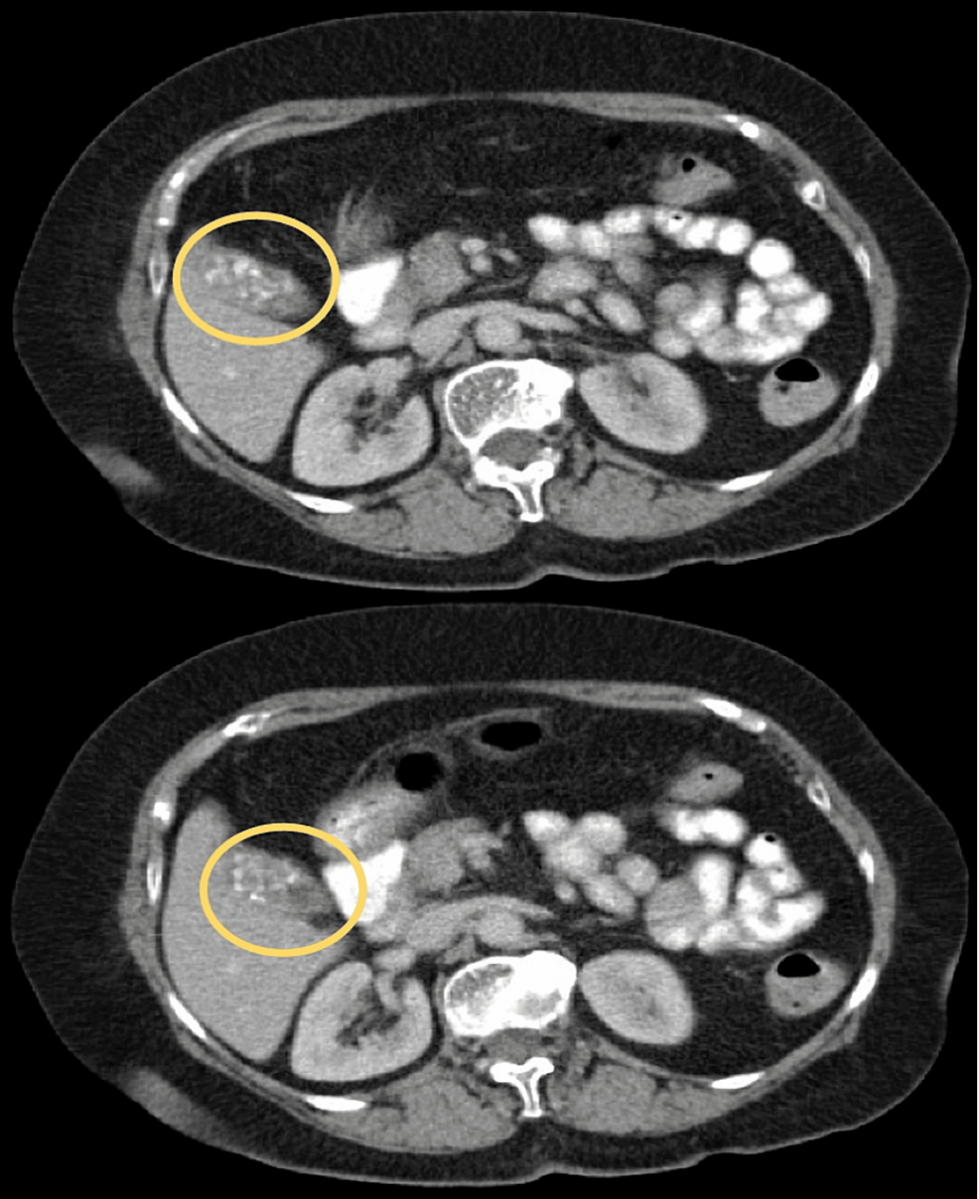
Pre-operative CT of the abdomen/pelvis demonstrating multiple impacted calcified gallstones. The yellow circles indicate multiple calcified gallstones that are impacted in the gallbladder.
